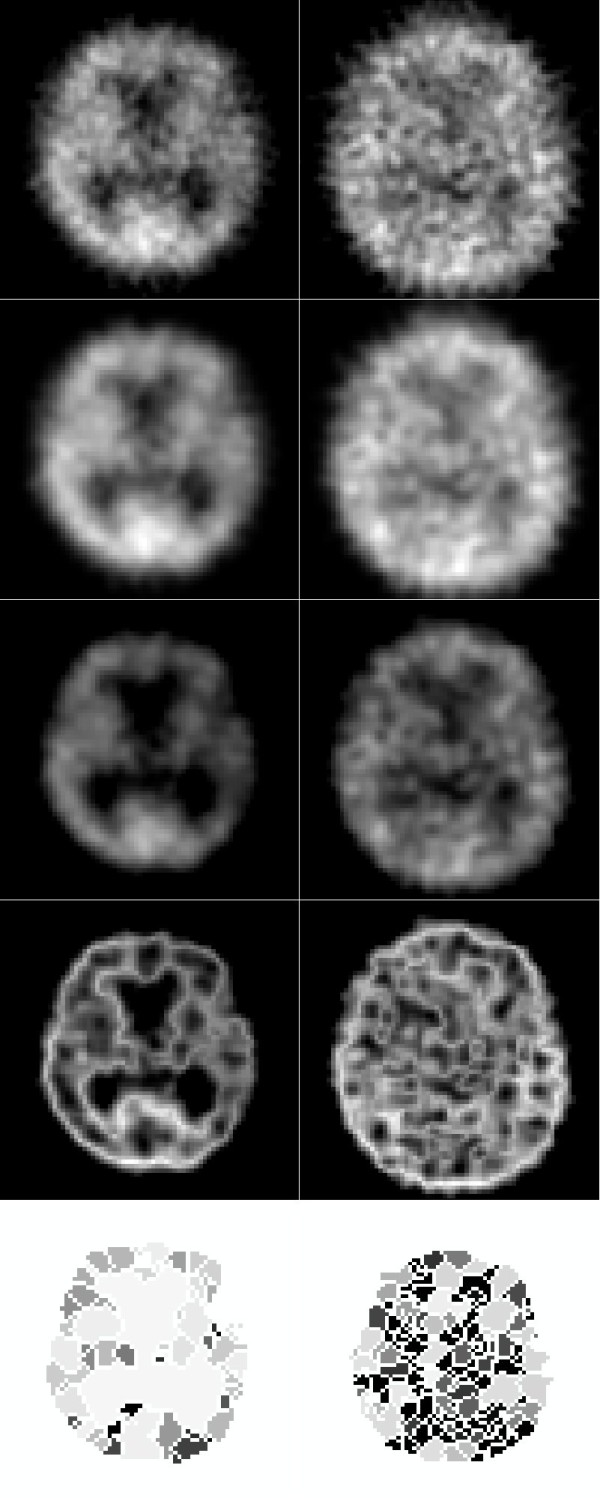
Processing steps for typical AD patients (left) and controls (right): original SPECT (1st - above), smoothing and normalization (2nd), thresholding (3rd), fuzzy edge detection (4th), watershed (5th - bottom).
